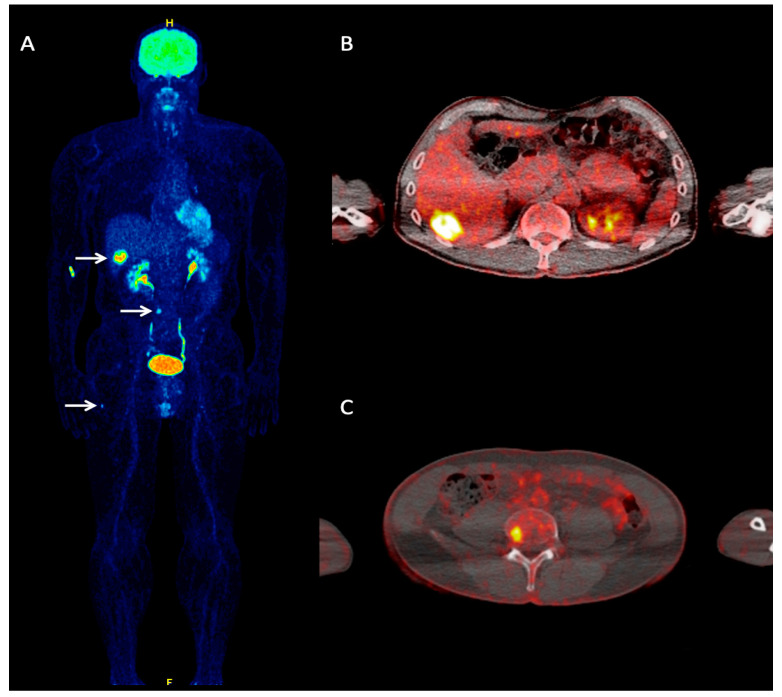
A 60-year-old Merkel cell carcinoma (MCC) patient referred to our department for staging purposes due to clinical suspicion of hepatic metastases. Whole-body ^18^F-FDG PET maximum intensity projection (MIP) (A) demonstrated foci of increased tracer uptake in the liver, lumbar spine, and right femur, corresponding to metastases (arrows). Transaxial, fused ^18^F-FDG PET/CT at the hepatic level (B) shows a focal site of increased tracer uptake in liver segment VI, corresponding to a hepatic metastasis. Transaxial, fused ^18^F-FDG PET/CT of the lower abdomen (C) shows pathologic tracer accumulation in the fourth lumbar vertebrae, representing an osseous metastasis.
